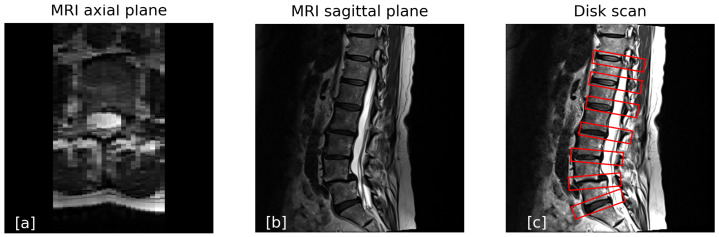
(a) The axial view of a T2 standard MRI. (b) The sagittal view of a standard MRI. (c) Sagittal view of the protocol for a skipping axial scan, in which only the intervertebral disks are scanned. The red boxes represent the parts that are scanned.
